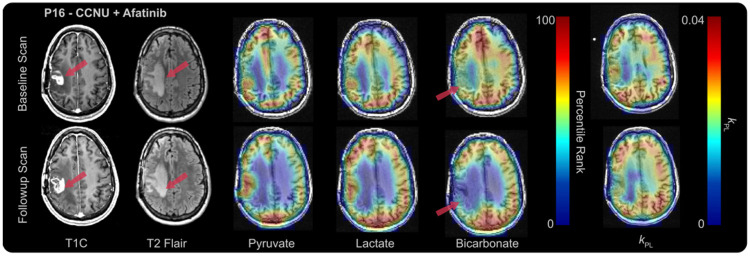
Representative slice for P16 at Pre-Tx (*top row*) and 2-month Post-Tx follow-up (*bottom row*). Post-contrast T1 and T2 FLAIR anatomical (*panels 1-2*), maps for Pyr%, Lac%, and Bic% (*panels 3-5*), and k_PL_ maps (panel 6) overlayed on the post-contrast T1 image. Arrows indicate T2 lesion.
